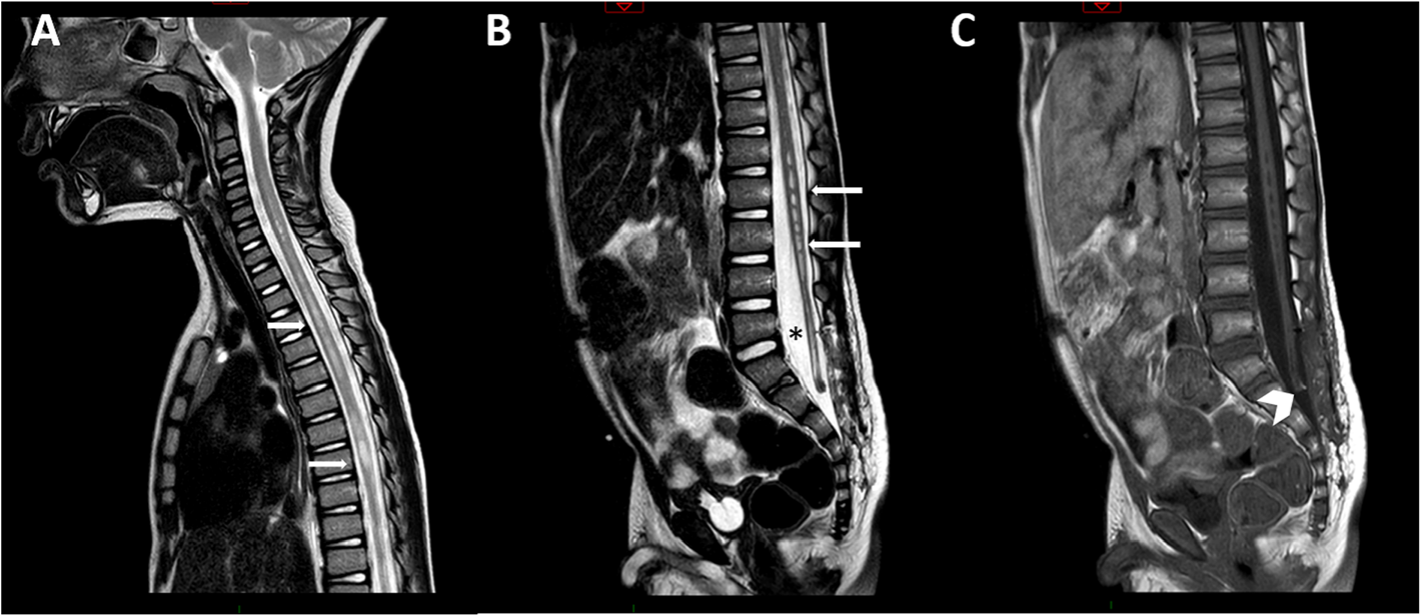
MRI scan of case #2 performed 3 months after surgery. The detethered spinal cord is now floating inside the dural sac (asterisk). The syringomyelia is significantly reduced on the sagittal T2 cervicodorsal (a, arrows) and lumbosacral MRI (b, arrows) scans. A very small remnant of the lipoma can be seen on the T1w sequence (c, head arrow)
